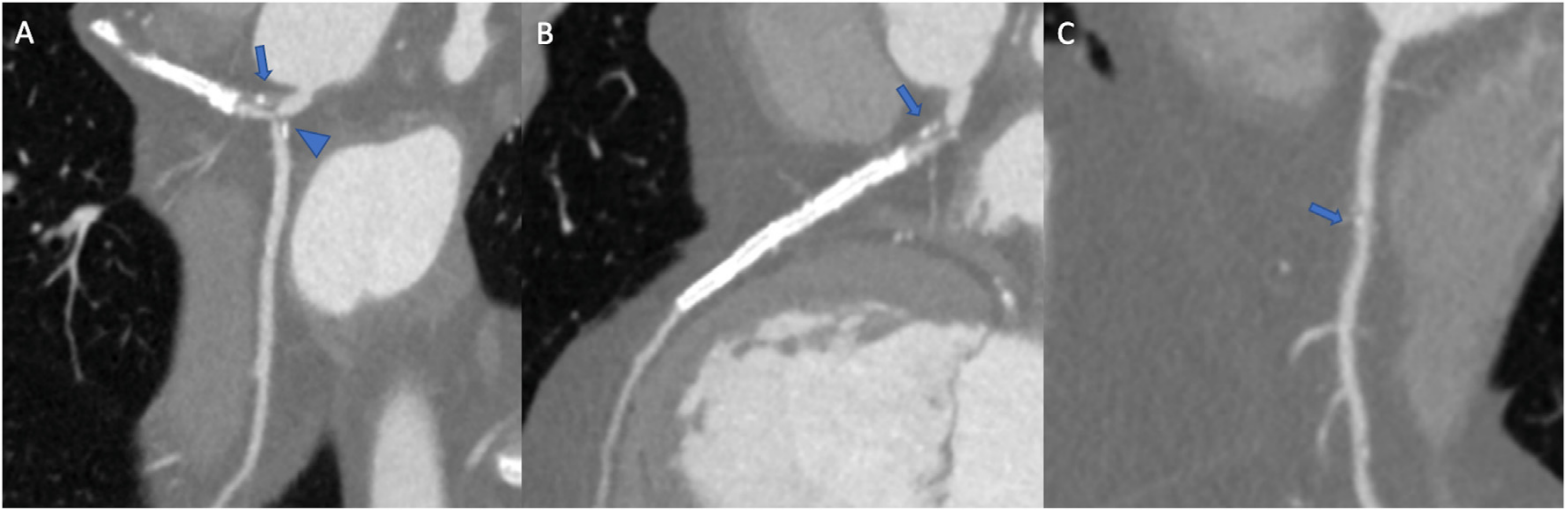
62-year-old male patient underwent coronary computed tomography angiography for chest pain in patient with previous PTCA and stent on left anterior descending artery. Left main coronary artery (A, arrow) and circumflex (A,arrowhead) show severe ostial mixed plaque, as well as left anterior descendant (B, arrow); the right artery only shows moderate calcific plaque at mid segment (C, arrow).
